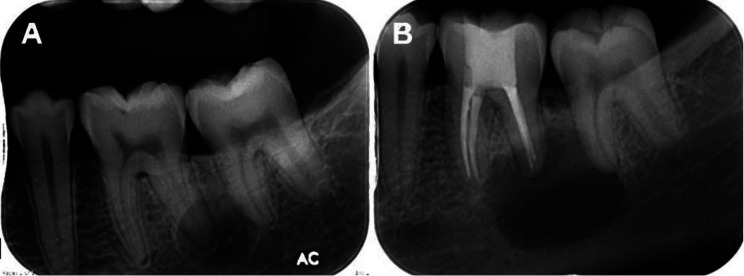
Periapical radiographs of an odontogenic keratocyst. A. A well-defined unilocular radiolucent lesion with corticated border is superimposed over the distal root of the lower left first molar. A thin radiolucent line of the periodontal ligament space of this root is clearly observed. B. The same lesion became larger despite root canal treatment for one year.
